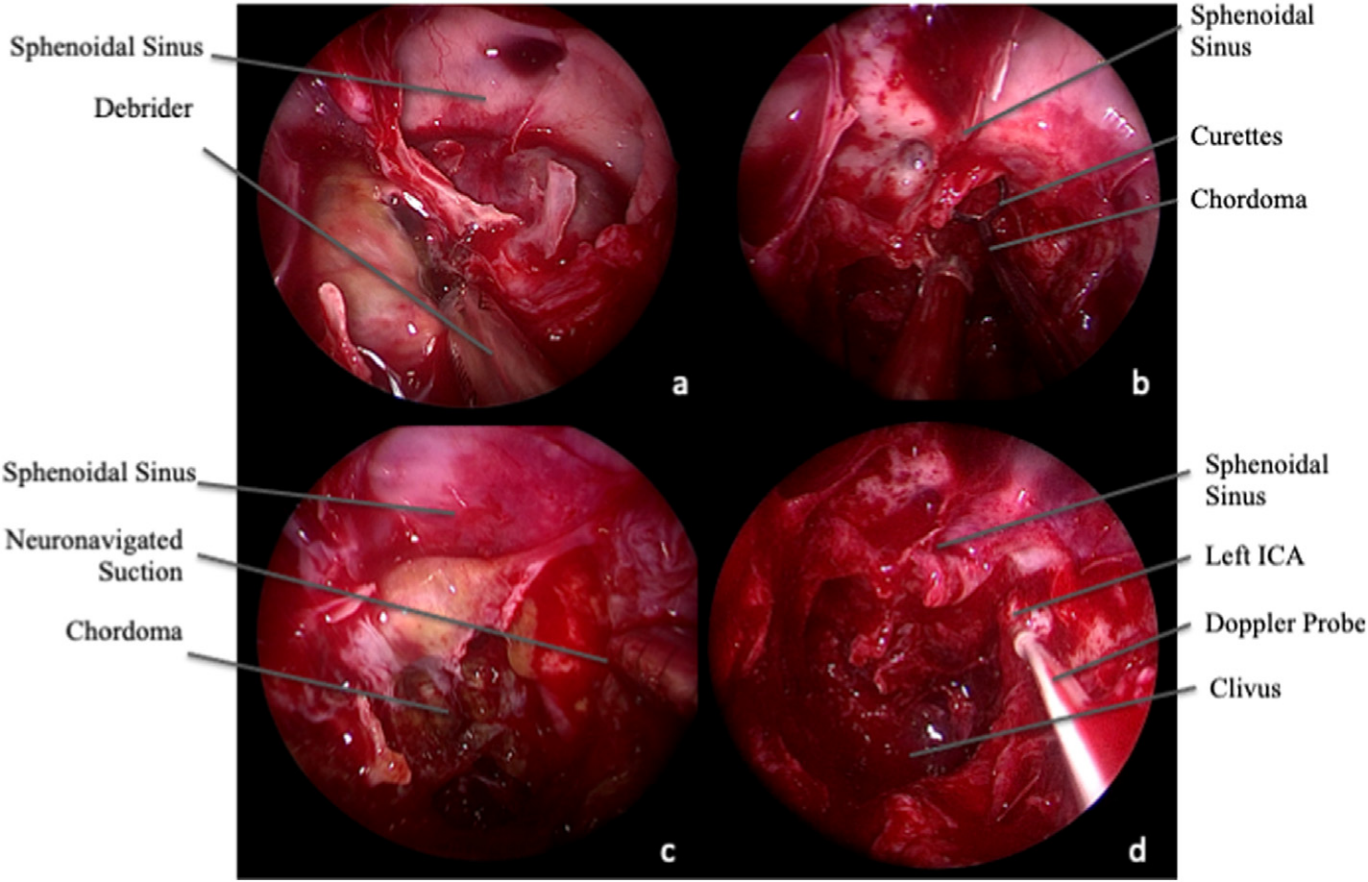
Intra-operative endoscopic image, 0° scope. Tumor removal. AA. The extracranial portion of the tumor can be resected with the debrider, which is particularly effective also in presence of scars from previous surgeries. B. In its intracranial portion, it is preferable to remove the tumor with curretes and suction, to reduce the risk of surgical complication. Ultrasonic aspirator can be also used, especially for firm CC. C and D. During tumor removal, it is necessary to check the location of the carotid arteries by neuronavigation (C) and intra-operative Doppler (D), reducing the risk of injuries to these vessels.
